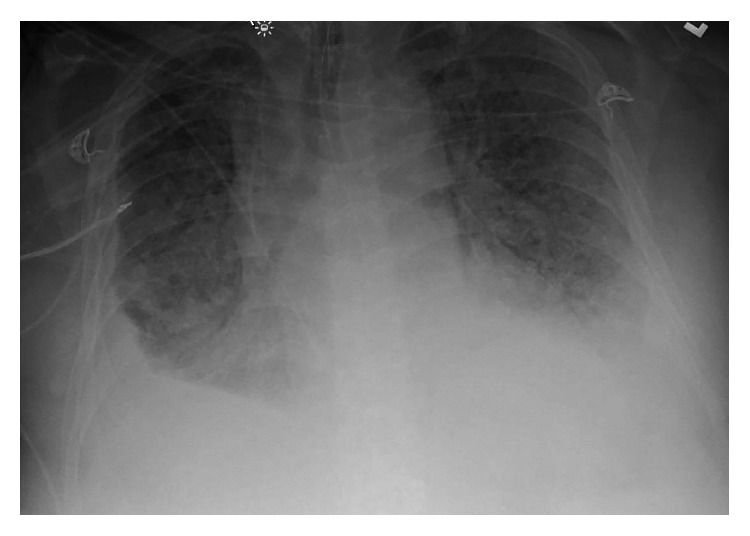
Chest radiograph showing bilateral diffuse infiltrates.
